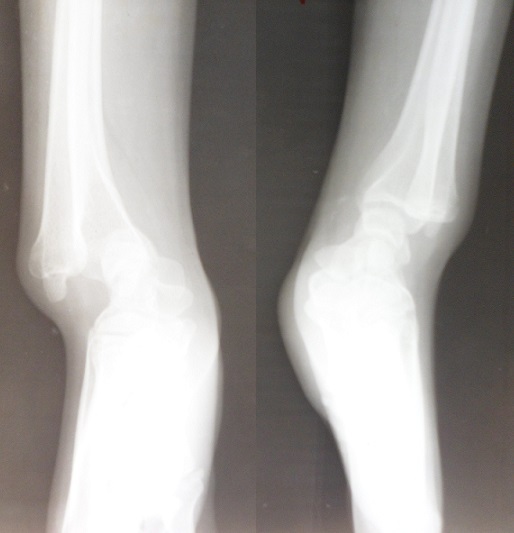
Radiographie comparative du poignet vue de profil
